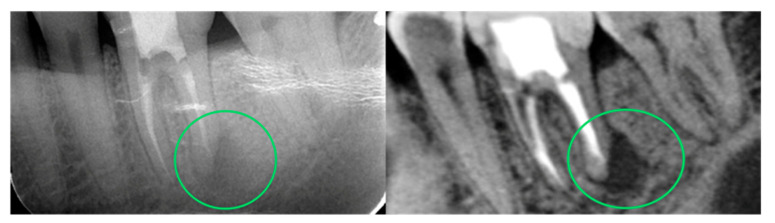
A comparison of conventional radiographs (left) and automated AI-enhanced radiographs (right) discretely outlying the anatomical features that can aid in improved diagnosis, prognosis, and accurate follow-up care. The highlighted area (green circle) show diagnostic enhanced region of interest.
